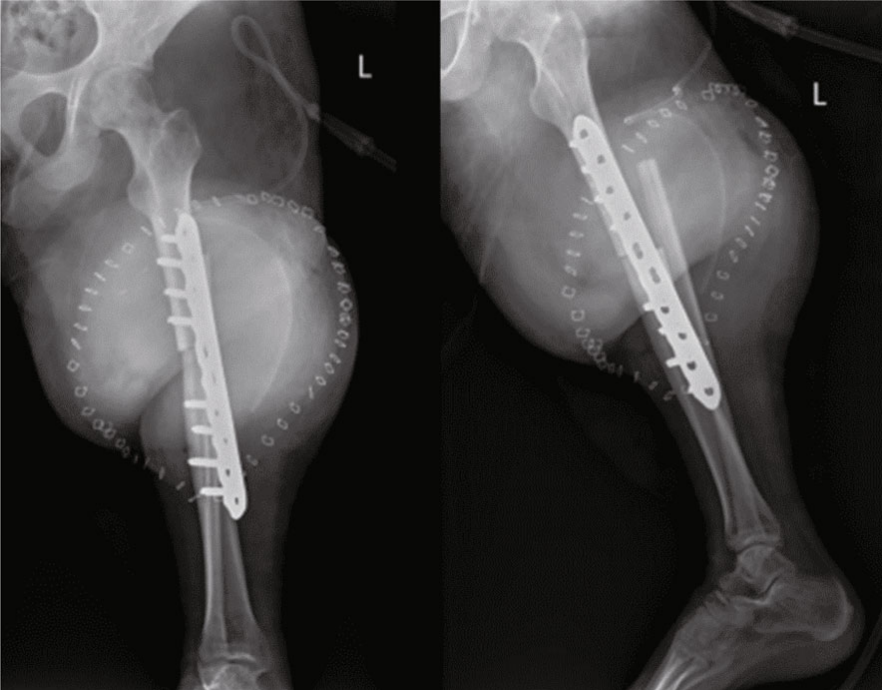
Postoperative X-rays of the surgical site.
